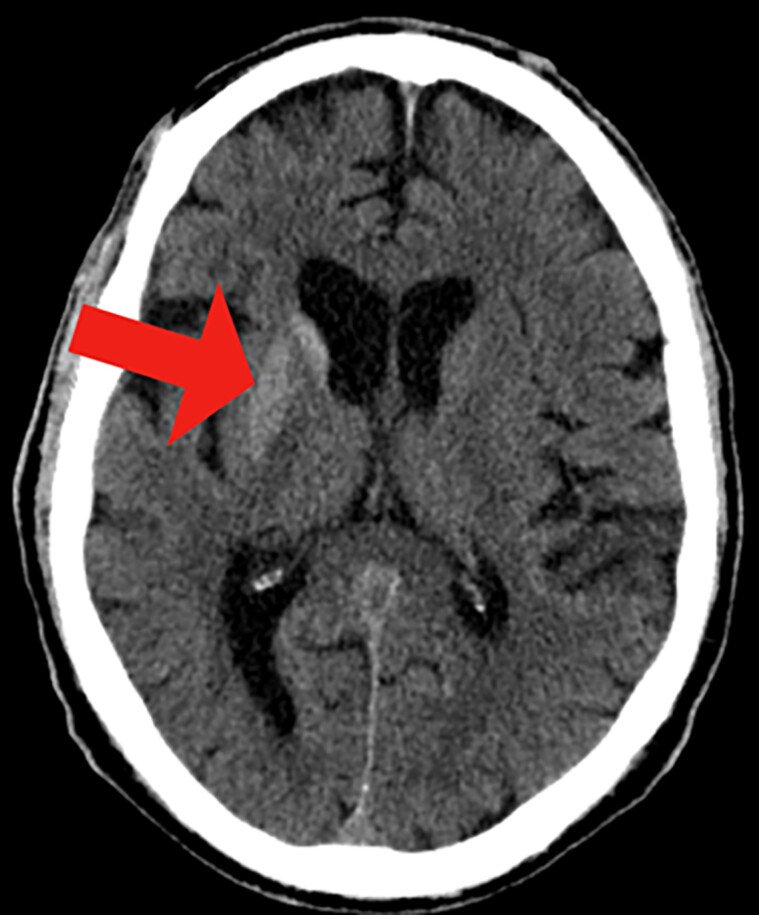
CT displaying unilateral hyperdensity of the right caudate and putamen (red arrow).
